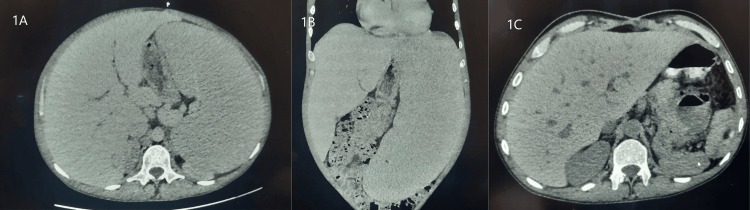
Huge splenomegaly in myelofibrosis patient A, B: axial and coronal view of abdominal CT scan shows huge splenomegaly; C: post splenectomy
